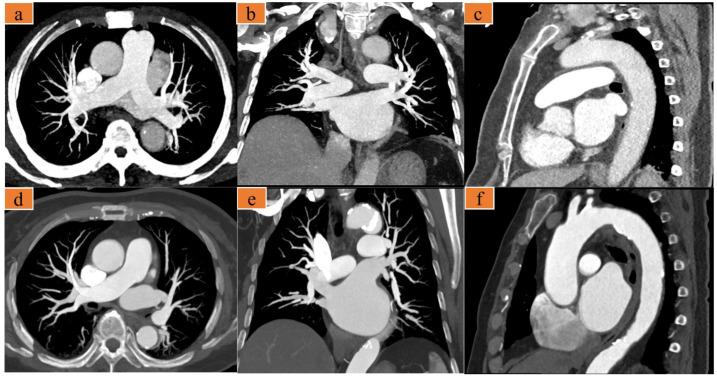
(a–c) A 75-year-old woman with chest pain, heart rate 77 bpm. Images were acquired with the optimized scanning scheme. (d–f) A 67-year-old woman presents to the emergency room with several days of chest pain, heart rate 66 bpm. Images were acquired with the traditional triple-rule-out computed tomography angiography (TRO-CTA). (a,d) are axial maximum intensity projection (MIP) images showing the pulmonary artery; (b,e) are coronal MIP images showing the pulmonary artery; and (c,f) are sagittal MIP images showing the thoracic aorta.
